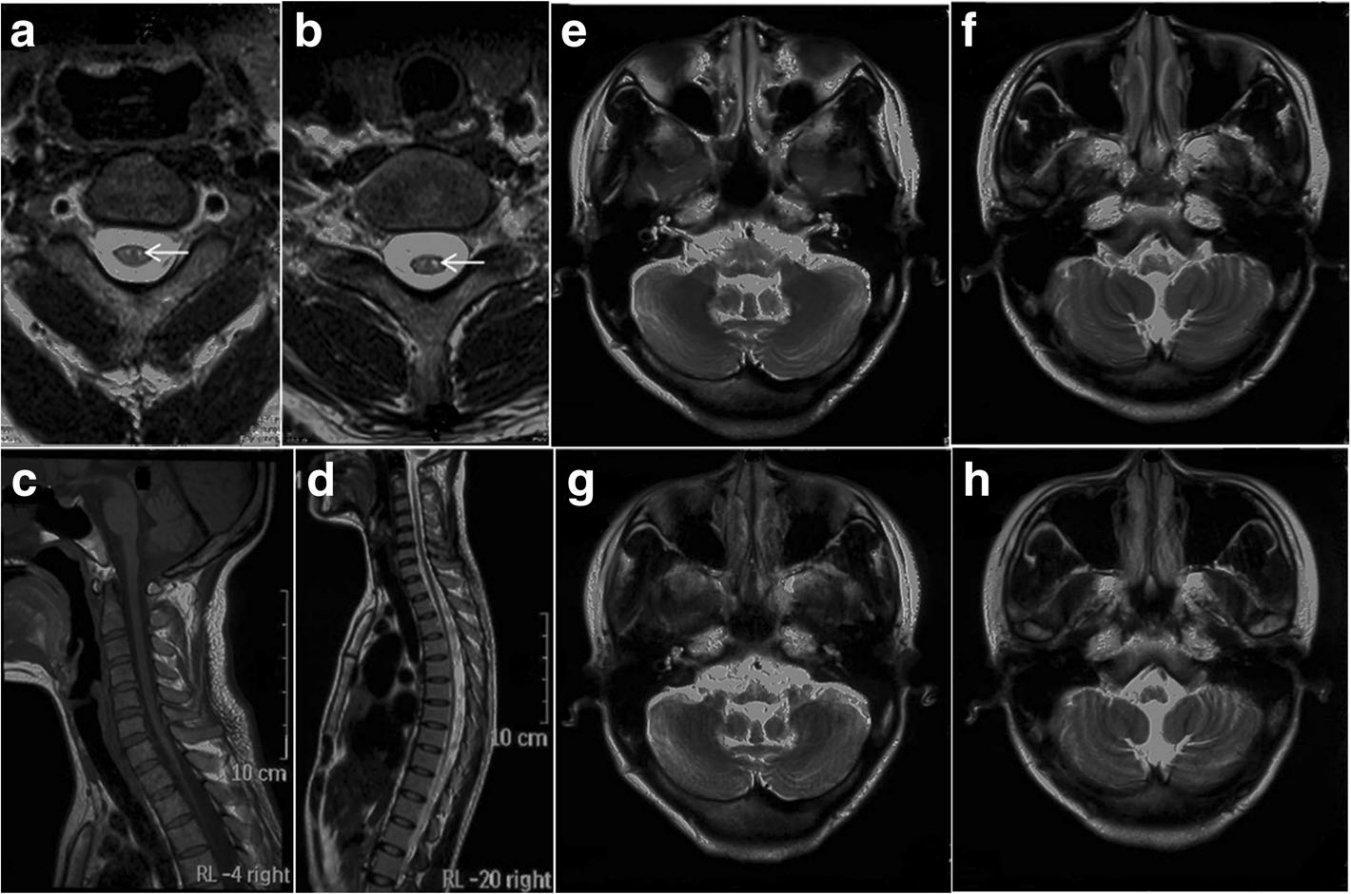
Axial spinal MRI showed hyperintensity involving the corticospinal tracts extends from the carotid 2 (a
